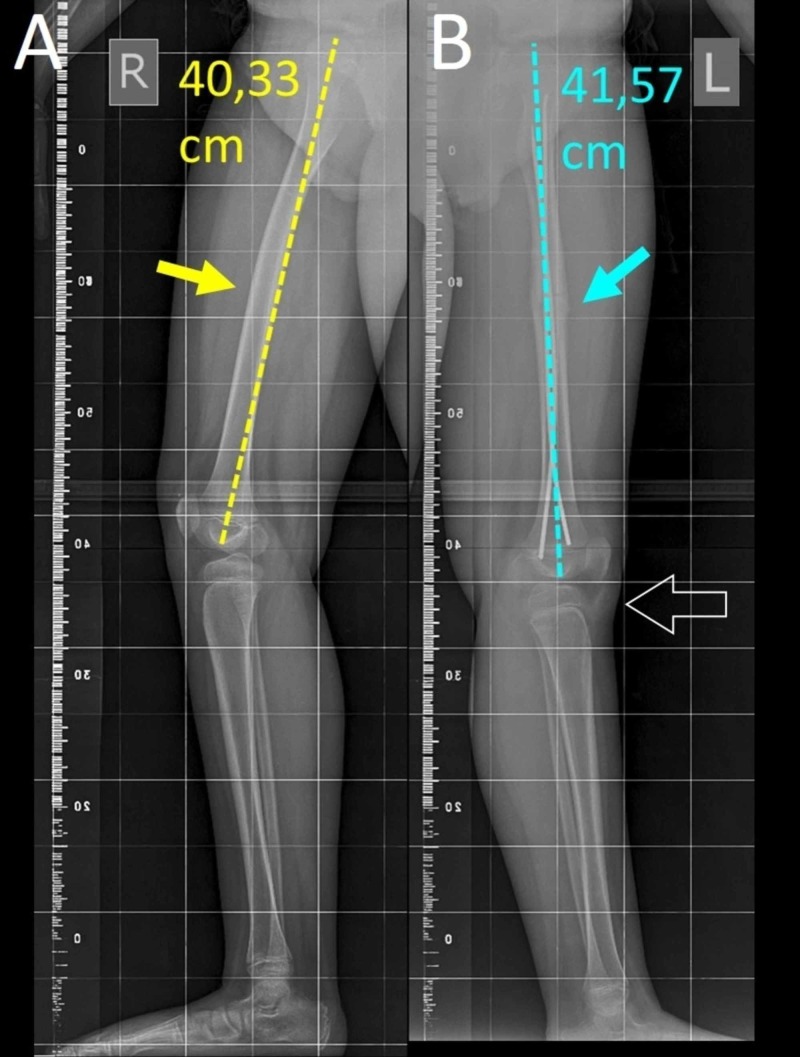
Long standing lateral X-ray view of the right (A) and the left (B) femur three months after surgery Limb length discrepancy with a longer left femur is obvious (+1.24 cm). A. Normal femoral antecurvatum of right femur (yellow arrow), B. The left femur is straightened due to loss of femoral antecurvatum (blue arrow). The left leg presents apparent (false) knee recurvatum secondary to loss of femoral antecurvatum (arrow outline).
